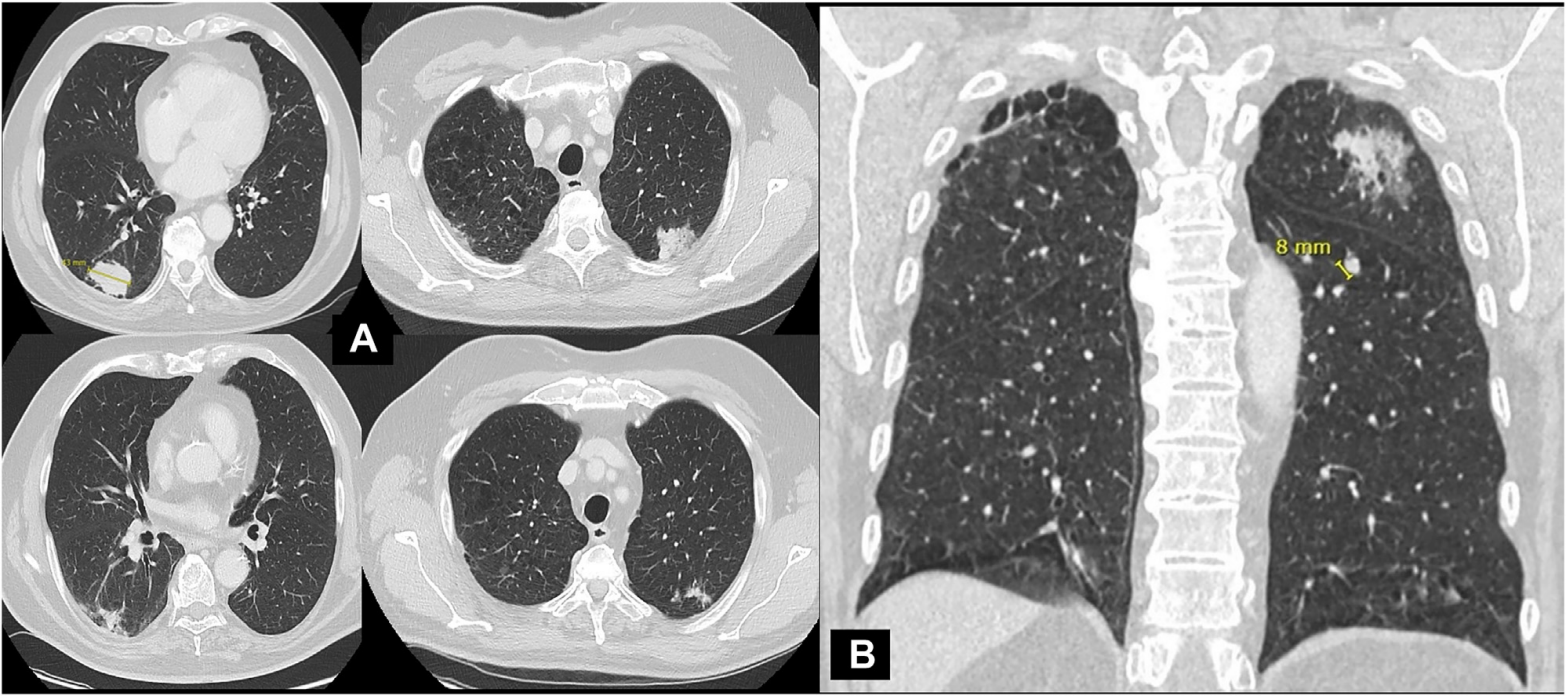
Computed tomography scans show (a) situation before (above) and after (below) 6 weeks of immunotherapy of right lower-lung and left upper-lung tumor with massive response of both tumors after immunotherapy and (b) frontal view of left upper-lung tumor and S6 nodule before immunotherapy.
